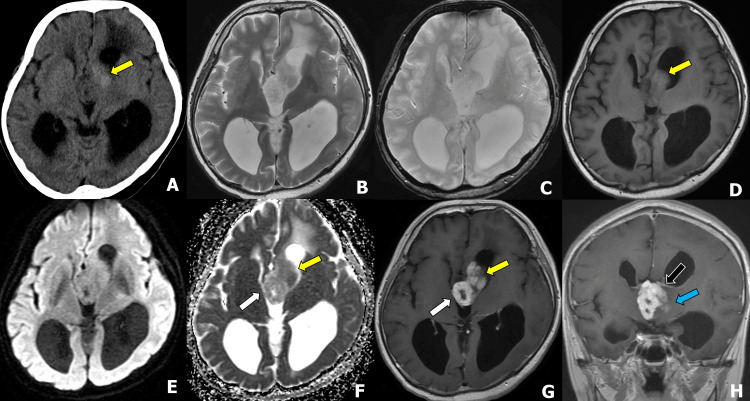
Preoperative CT and MRI one month after the initial visit. (A) Axial computed tomography (CT), (B) T2-weighted image (T2WI), (C) T2*WI, (D) T1WI, (E）diffusion-weighted image (DWI), (F) apparent diffusion coefficient (ADC) map, (G) axial postcontrast T1WI, and (H) coronal postcontrast T1WI. The tumor consisted of two following components: the ventral component appeared dense on CT, slightly hyperintense on T1WI, demonstrated contrast enhancement on postcontrast T1WI, and had a decreased ADC value (F; yellow arrow). The dorsal component showed ring enhancement on postcontrast T1WI and an increased ADC value in the solid component (E and F; white arrows). In DWI, both components showed signals close to the cerebral white matter, and no marked high signals were observed. No hemorrhage or calcification was observed on T2*WI (C). The tumor predominantly occupies the third ventricle and left Moro foramen, located beneath the thalamostriate vein (H; black arrow), and appears contiguous with the brain parenchyma (H; blue arrow).
